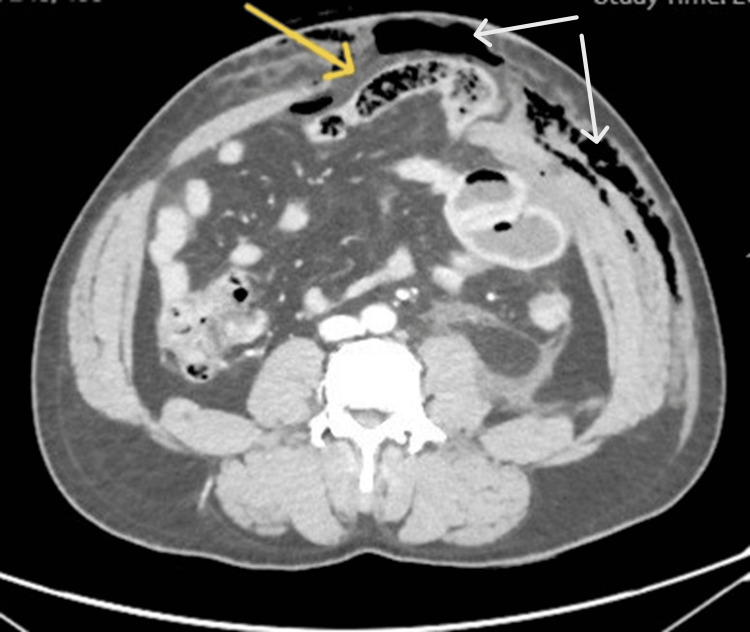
Computed tomography (CT) scan The image shows an abdominal wall defect with a herniating bowel loop (yellow arrow) and subcutaneous emphysema (white arrows), suggestive of bowel perforation.
